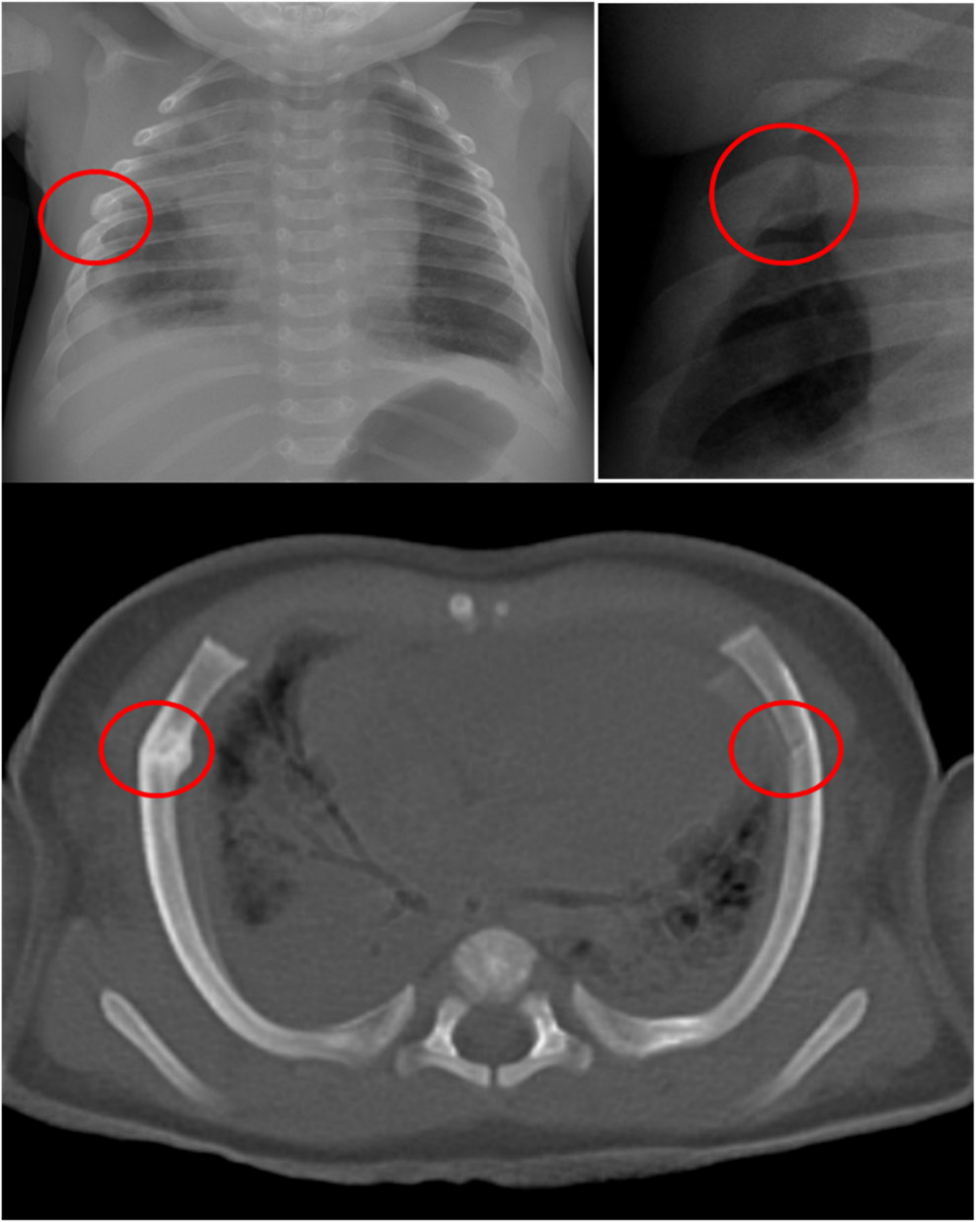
PMCT for fractures. PMCT is becoming particularly useful at detecting rib fractures. An example is given of a 4 month old girl with a rib fracture on the right, difficult to identify on the frontal chest radiograph (a), easier to see on the oblique view of the left sided ribs (b), and very easy to identify on axial 3D reconstructed PMCT (c). PMCT confirms that there are bilateral anterolateral fractures, in a typical location resuscitation related injuries, and there were no other signs of injuries in this child. Fresh anterolateral fractures are highly likely to be related to resuscitation if there are no other associated injuries
